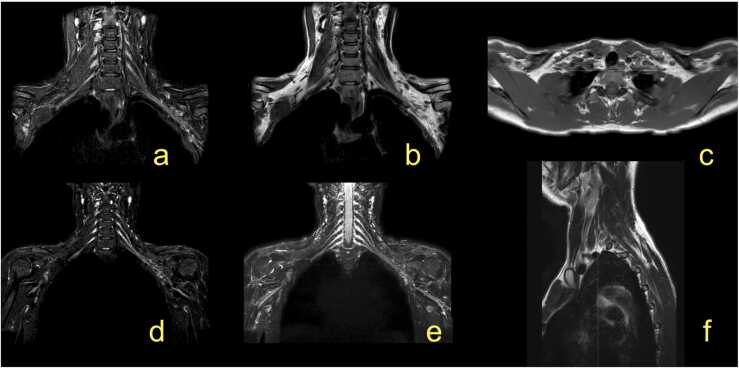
The magnetic resonance imaging protocol used at the authors' institution. (a) T2-weighted modified DIXON sequence with fat suppression, (b) T2-weighted modified DIXON sequence without fat suppression, (c) T1-weighted turbo spin-echo (TSE) sequence, (d) 3D neurography, (e) neurography MIP 3 mm, (f) T2-weighted TSE sequence.
